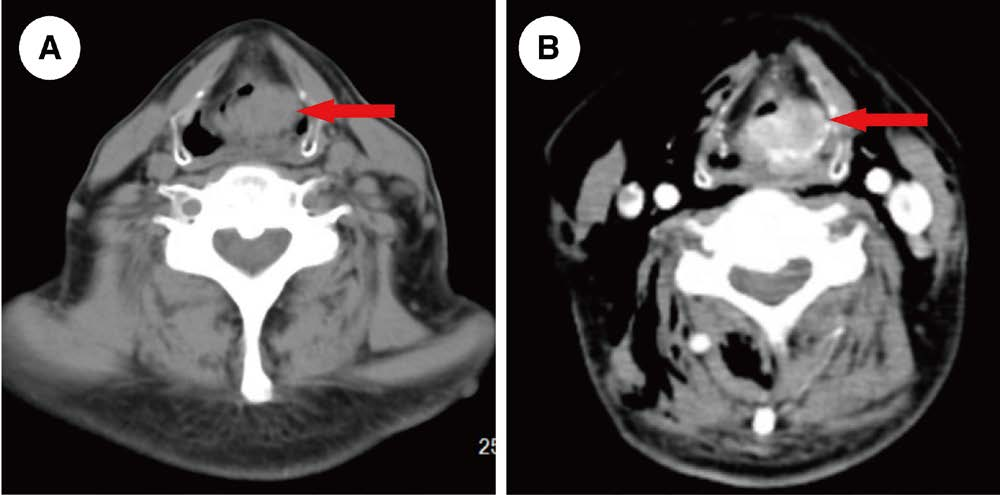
Enhanced CT showed a shadow of soft tissue density in left supraglottic region with an indistinct border with surrounding tissues and uneven enhancement (red arrow). CT = computed tomography.
